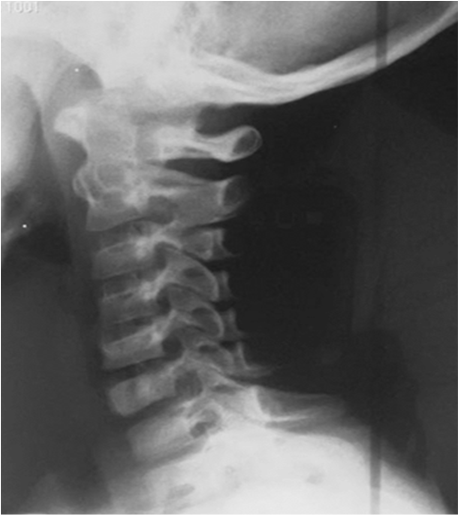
Radiographie du rachis cervical profil d’une patiente de 7 ans: interprétée normale
